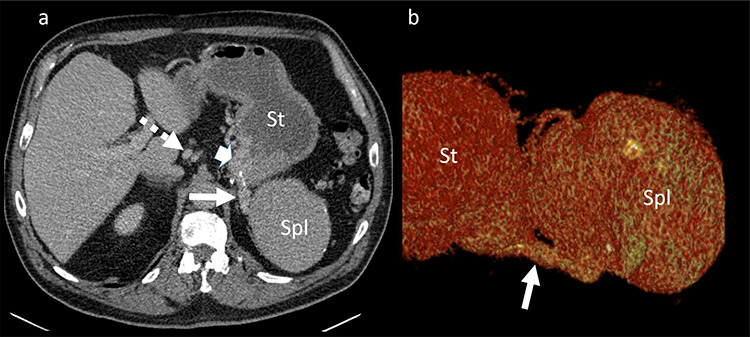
Portal venous phase CT of the upper abdomen (a) with a 3D reconstruction (b); note the gastric varices (arrowhead) and dilatation of short gastric veins (solid arrow) and coronary vein (dashed arrow); St: stomach; Spl: accessory spleen.
